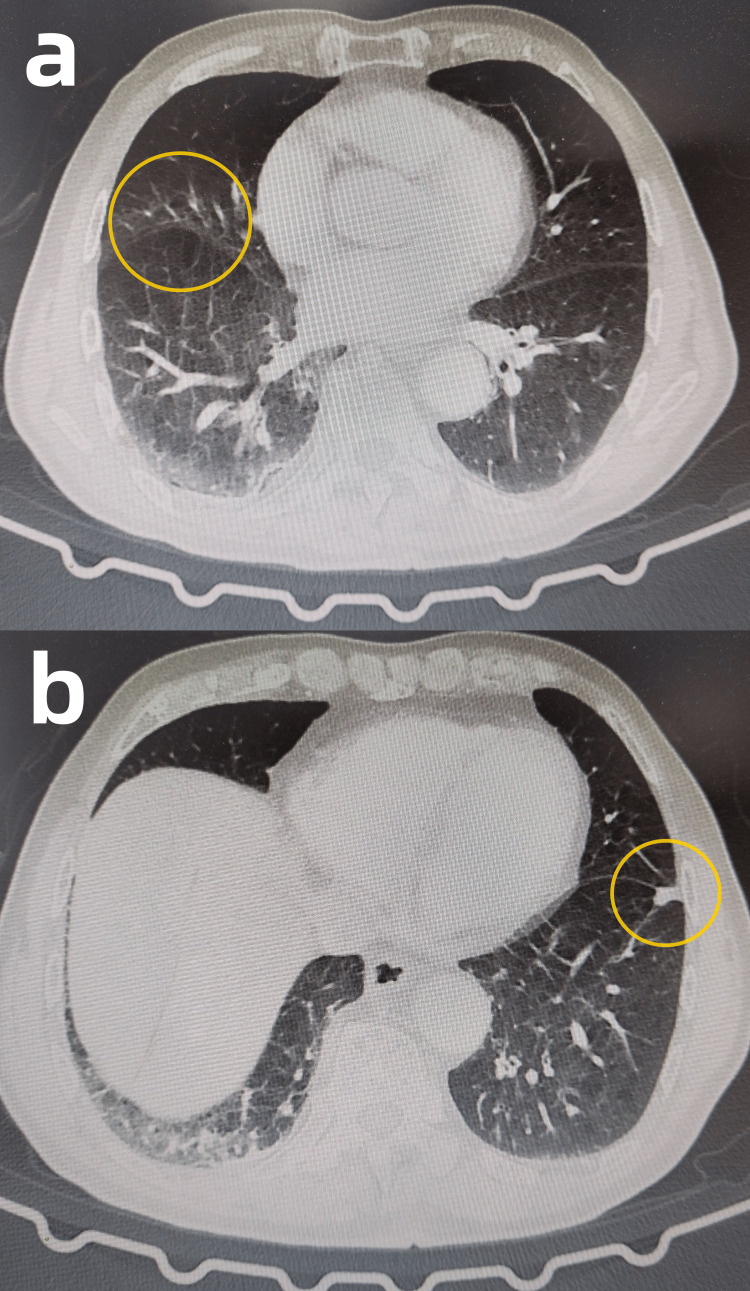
Axial CT scan of the chest (a) The yellow circle indicates pulmonary emphysema and mild inflammation in the right middle lobe. (b) The yellow circle indicates a solid nodule in the anteromedial basal segment of the left lower lobe. CT: computed tomography
